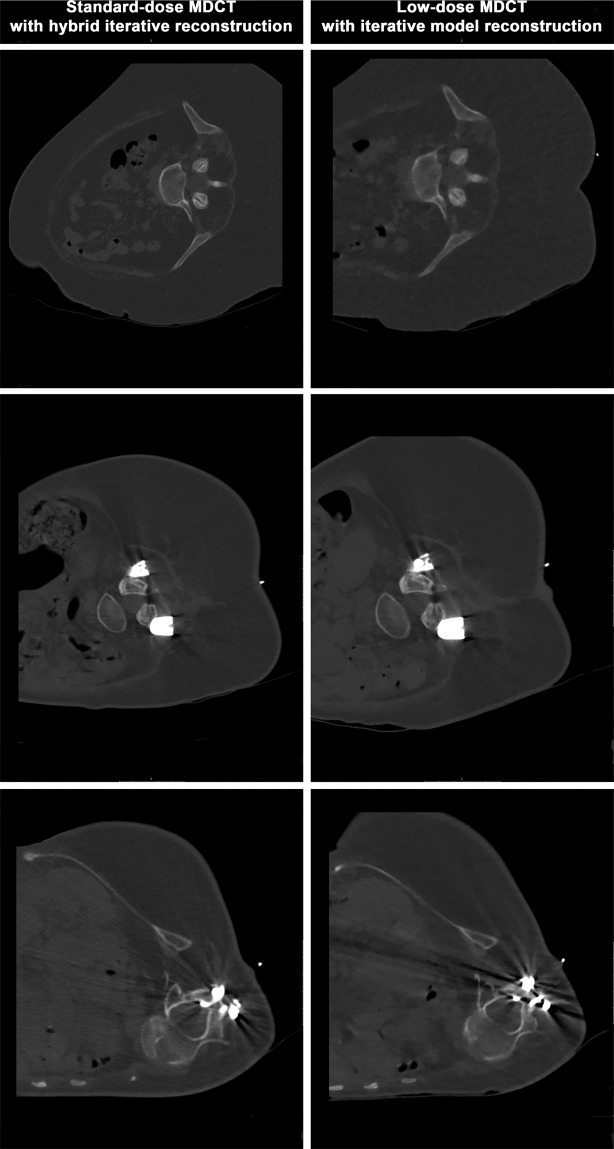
Standard- and low-dose scans for procedure planning of intrathecal nusinersen administration. This figure shows three exemplary patient cases (one patient case per horizontal column, two axial slices per patient case) using both standard-dose and low-dose scanning by multi-detector computed tomography (MDCT). Image reconstructions for standard-dose scans were performed using a hybrid iterative reconstruction algorithm (iDose4; Philips Healthcare, Best, The Netherlands). Low-dose scans were reconstructed with iterative model reconstruction (IMR; Philips Healthcare, Best, The Netherlands).
